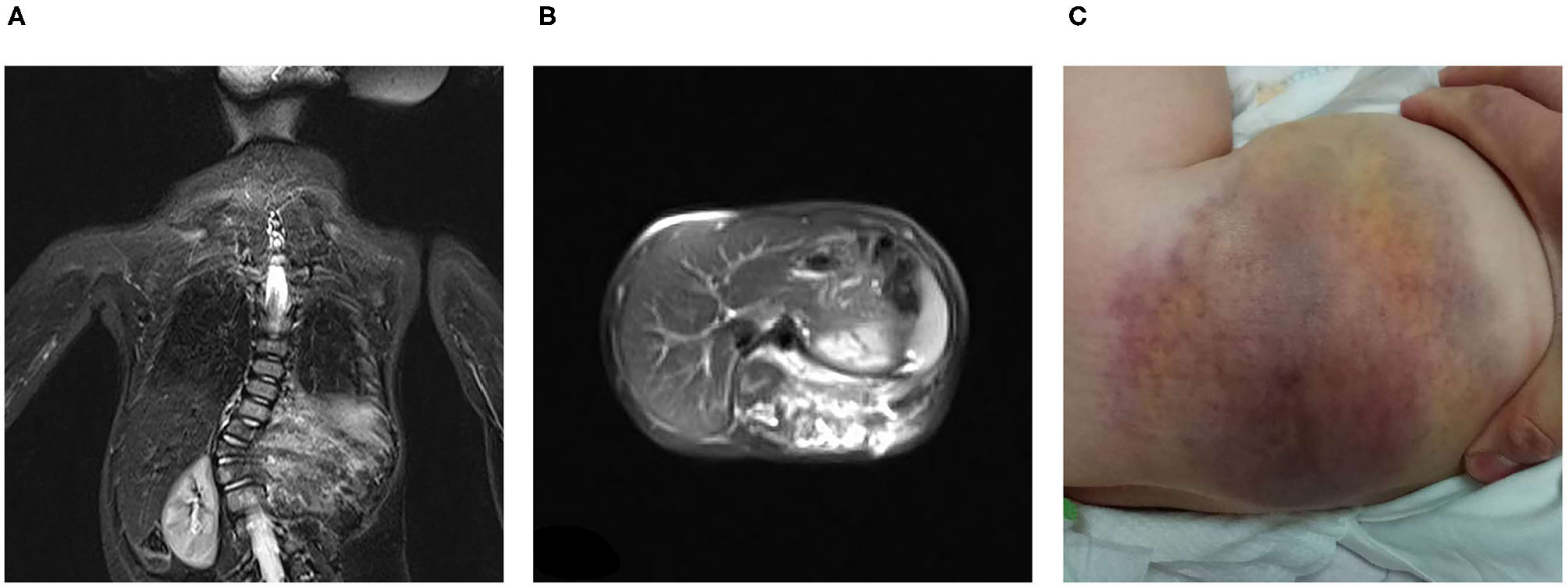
KHE in Patient 6: The MRI showed that large mixed signal mass involved the left lumbar and back, and T7-L4 vertebrae and paraspinal tissue from coronal (A) and sagittal position (B), and we can see that the skin on the left waist and back was involved (C).
